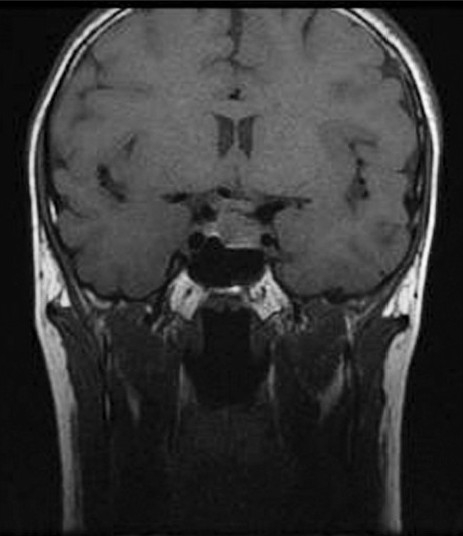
T1-weighted coronal MRI scan showing a pituitary tumor in a child
